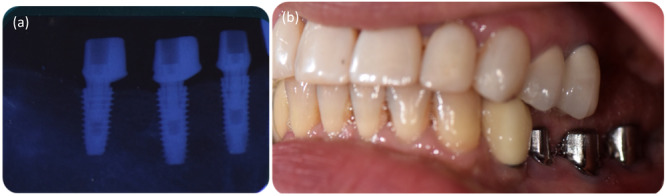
The definitive abutments at the time of implant insertion. (a) Radiographic view, (b) Intraoral view.
